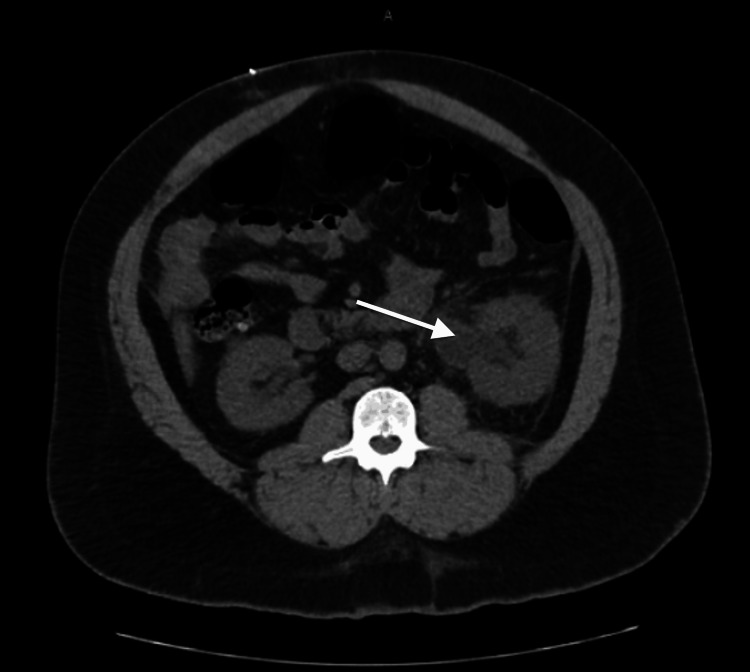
Left-sided hydronephrosis, transverse non-contrast CT of the abdomen.
